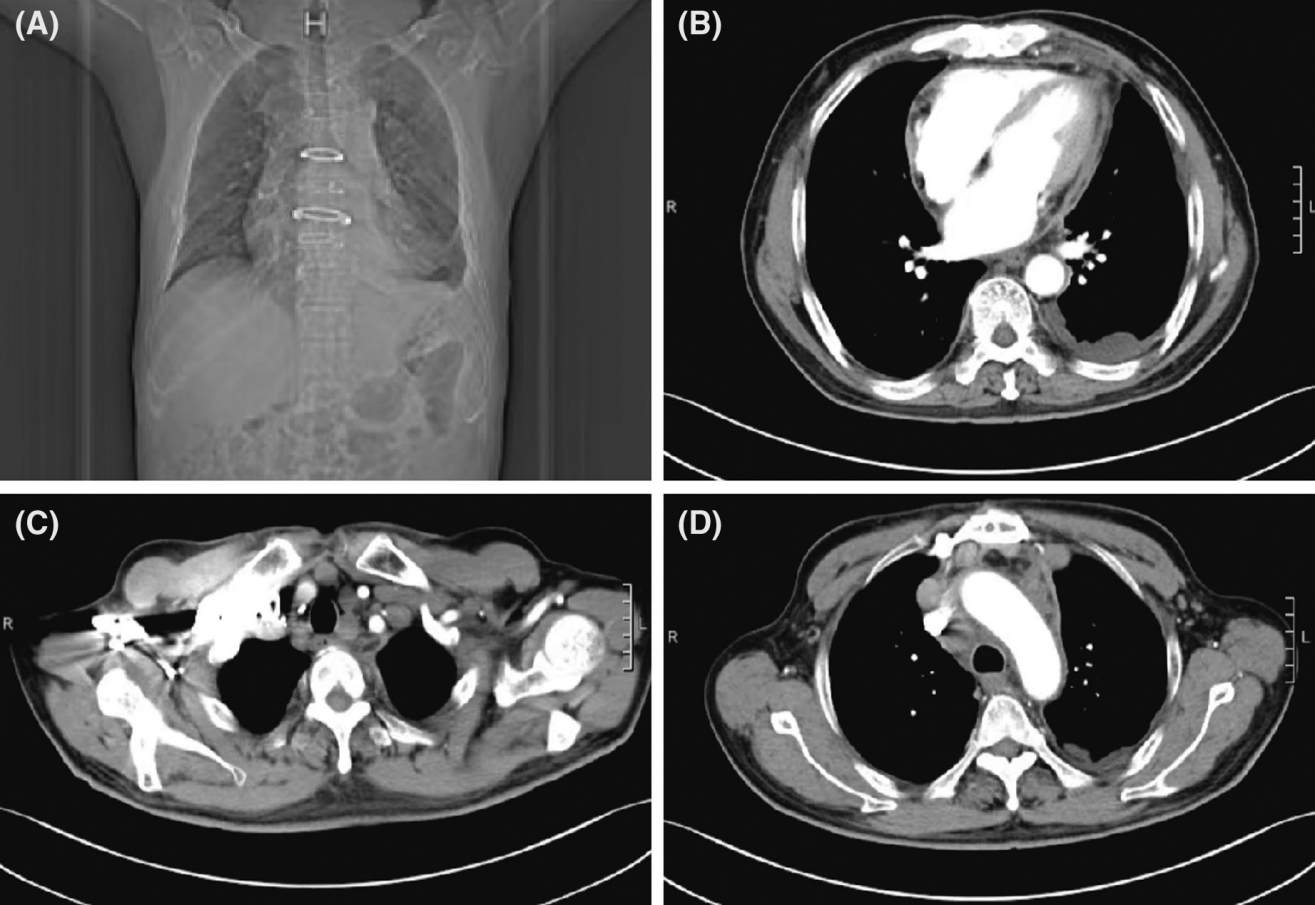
Postoperative CT (A) Sternal internal fixation. (B) Bilateral pleural thickening, left lung insufficiency, pericardial thickening combined with a small amount of pericardial effusion. (C) Enlargement of lymph nodes in the superior and inferior clavicular fossa. (D) Mediastinal lymph node enlargement.
